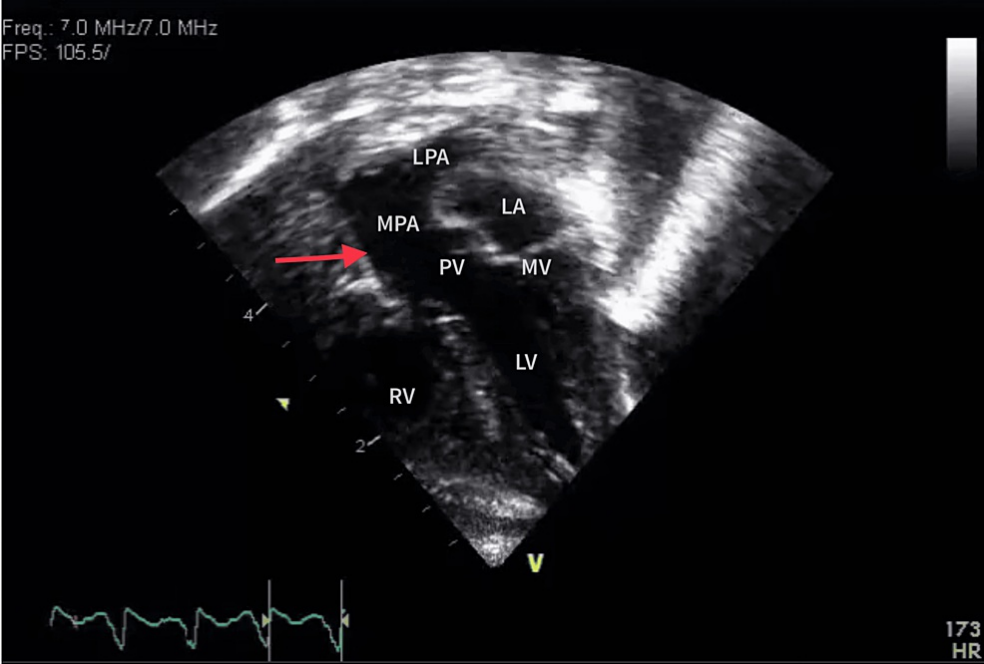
Apical five-chamber view The red arrow shows the pulmonary artery originating from the left ventricle. RV, right ventricle; LV, left ventricle; MV, mitral valve; PV, pulmonary valve; MPA, main pulmonary artery; LPA, left pulmonary artery; LA, left atrium
